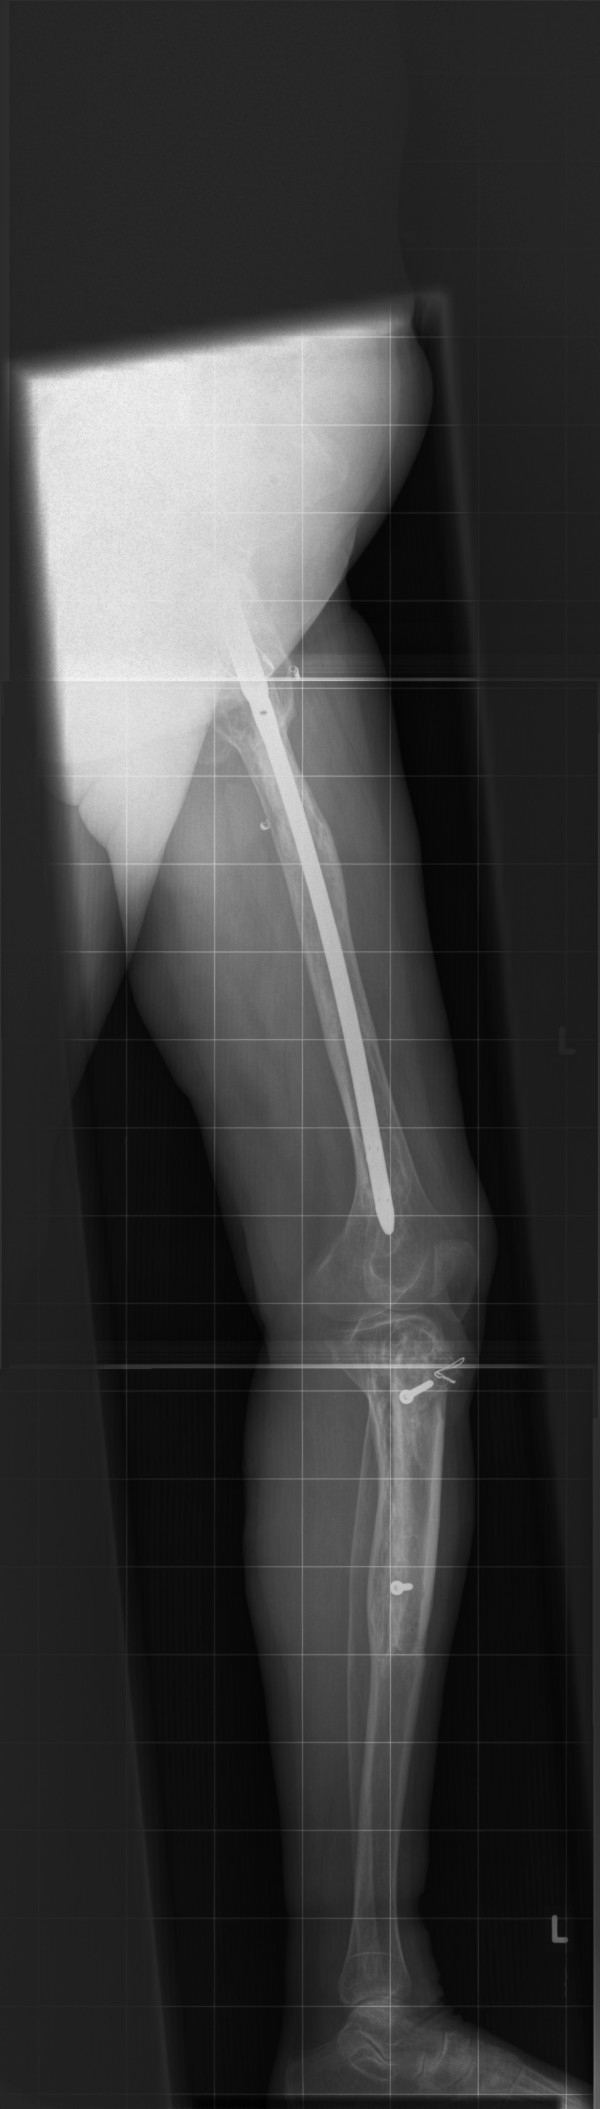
Lateral X-ray of the whole left leg. This X-ray shows the situation after fibrous dysplasia excision of the left proximal femur and left tibia.
